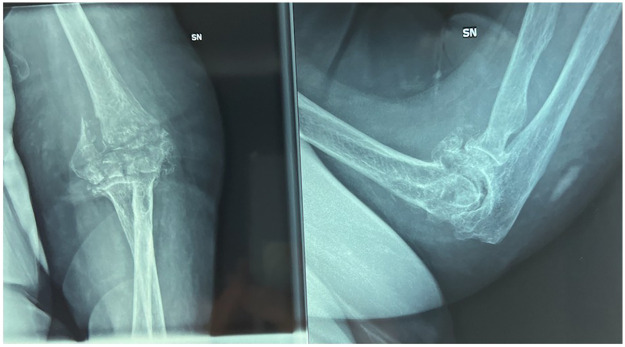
Preoperative anteroposterior and lateral radiographs of the elbow.
